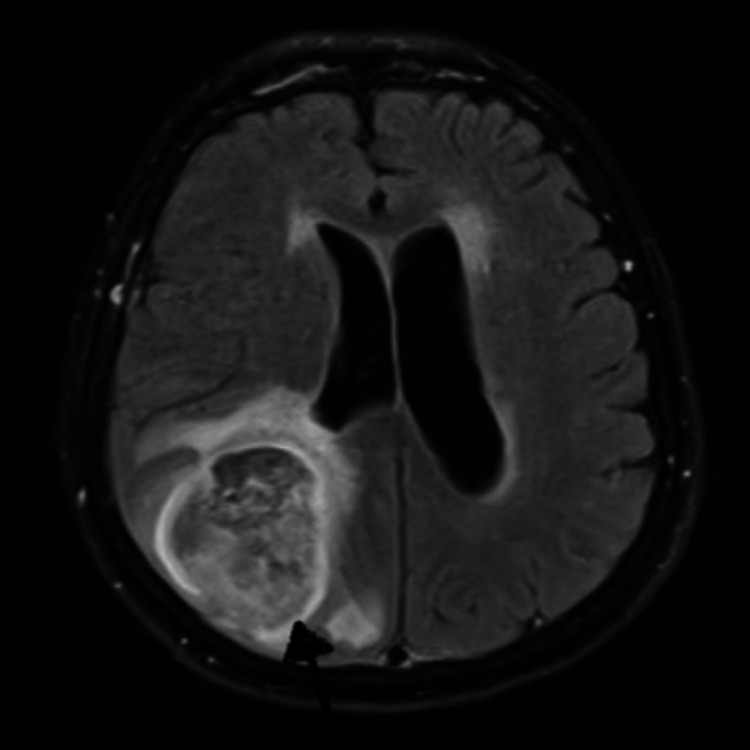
MRI (Axial View) Showing the Homogenous Lesion in the Right Parietal Lobe
